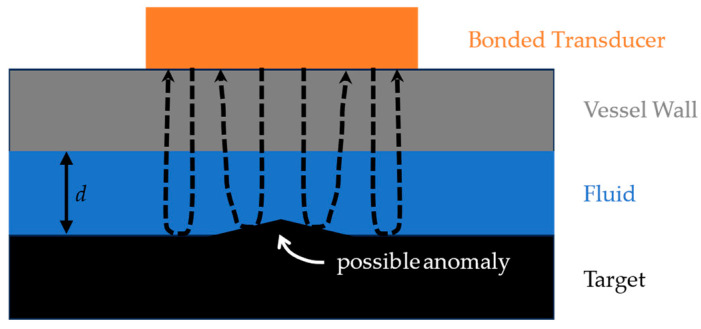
Pulse-echo ultrasound immersion test configuration.
